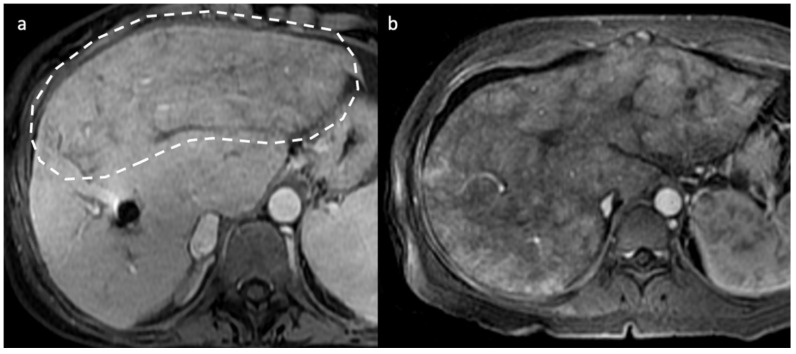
In subacute or chronic BCS morphological changes and fibrosis occur in the liver and regions of hypoperfused parenchyma can still be seen with the so-called ‘‘mosaic’’ enhancement pattern on both CT and MR as a sign of sinusoidal dilatation. Axial portal venous phase (a) and arterial phase (b) MR images show two different cases of partial (white circle) and diffuse mosaic pattern in the liver, characterized by reticular enhancement.
